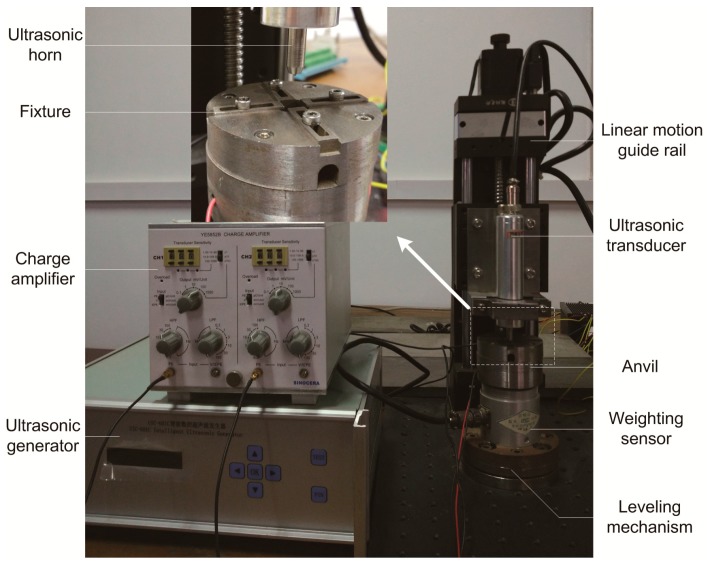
Ultrasonic bonding test bench.
